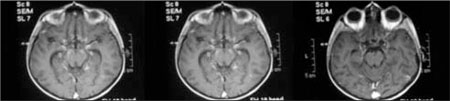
The tuberculous granulomas seen in the basal cisterna in contrast and non-contrast T1-weighted magnetic resonance imaging (MRI) in cross sections
